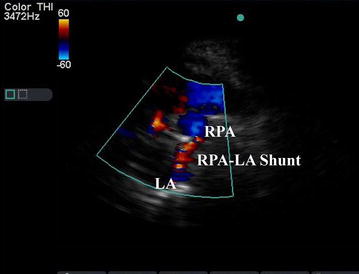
Transthoracic echocardiography showing shunt. *RPA* right pulmonary artery, *LA* left atrium
